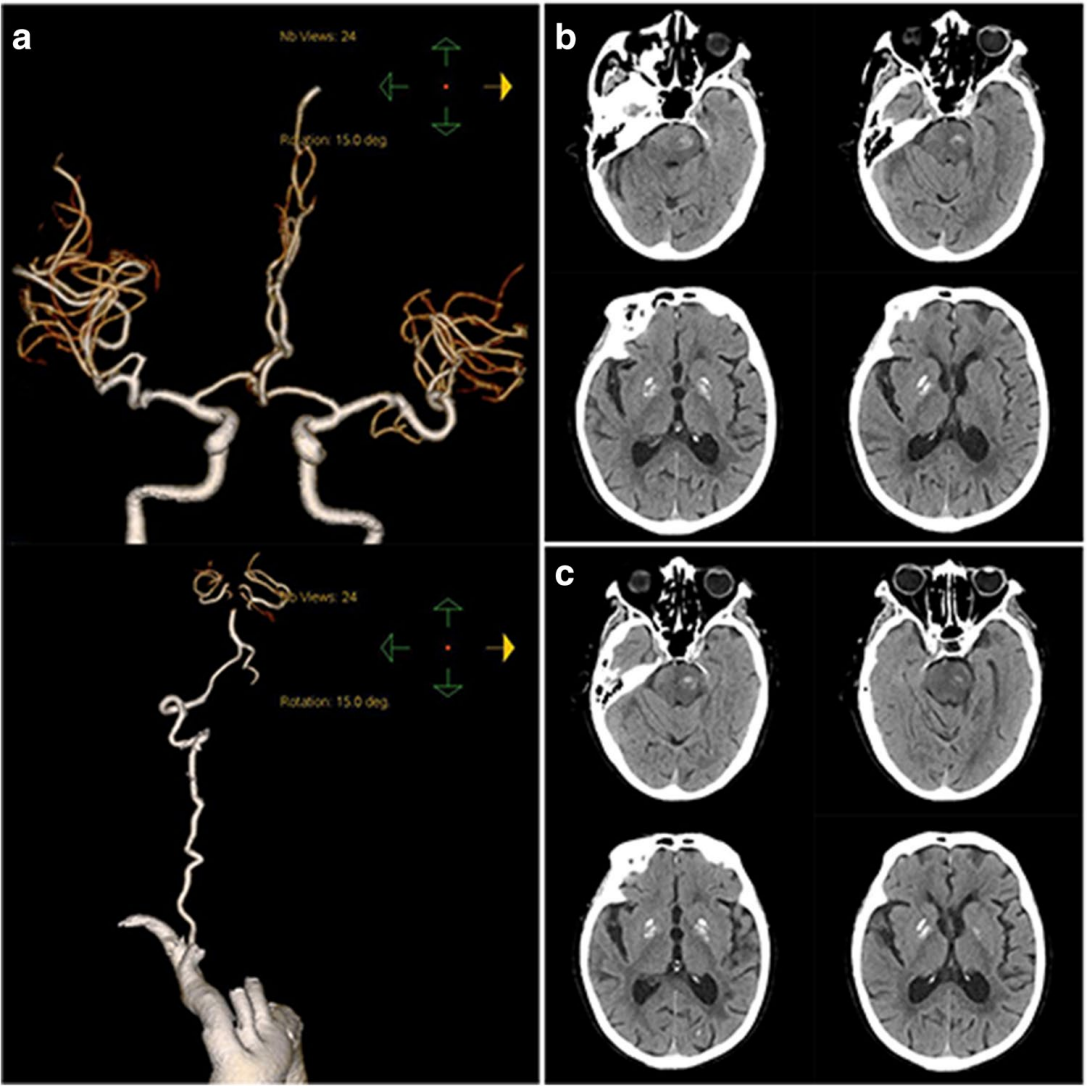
Imaging of patient with the second stroke. a Head and neck CTA on the 12th day after admission: the left vertebral artery and the terminal of the basilar artery were not visualized as before; the basilar artery and the origin of bilateral posterior cerebral arteries were not visualized locally. b Head CT on the 13th day after admission: small patchy hemorrhages in the left occipital lobe and pons. c Head CT on the 15th day after admission: compared with the CT on the 13th day after admission, the hematoma did not change significantly
